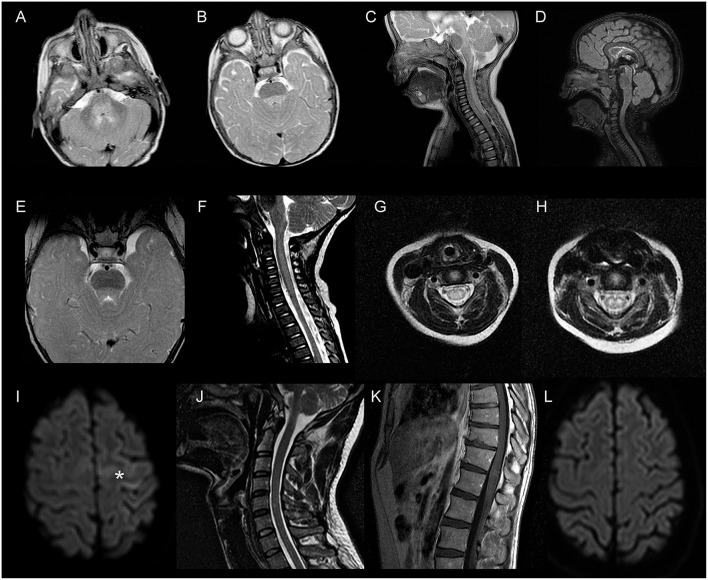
Brain and spinal cord MRI findings. Patient 1 – (A–C): dorsal brainstem and cervical hyperintensities on T2-weighted images. (D): sagital FLAIR image shows disappearance of previous spinal cord hyperintensity. Patient 2—(E–G): dorsal brainstem and cervical diffuse and poorly defined T2-hyperintensities during the acute phase; (H): hyperintense T2 signal of the anterior horn of the cervical spinal cord one week later. Patient 3—(I–K): left frontal cortical hyperintensities (asterisk) in diffusion weighted images (DWI); cervical and lumbosacral hyperintensities on T2-weighted imaging with enhancement on post-gadolinium image. (L): axial DWI at 3 months shows resolution of the previous alterations.
